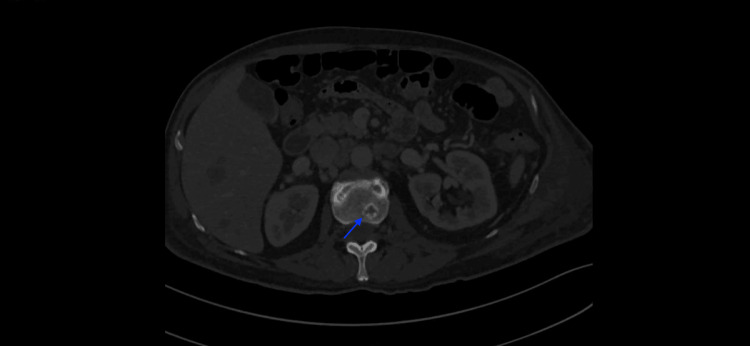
Abdominal CT scan The image shows bone metastases (blue arrow). CT: Computed tomography.
